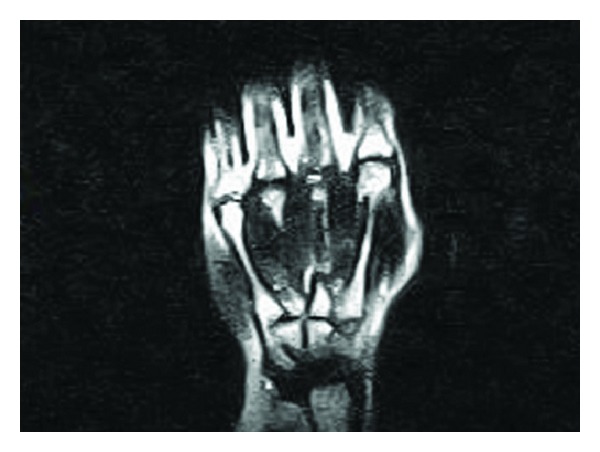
MR image of the volunteer with RFID tag attached to the wrist (imaging sequence: T_1_—Spin echo Coronal).
